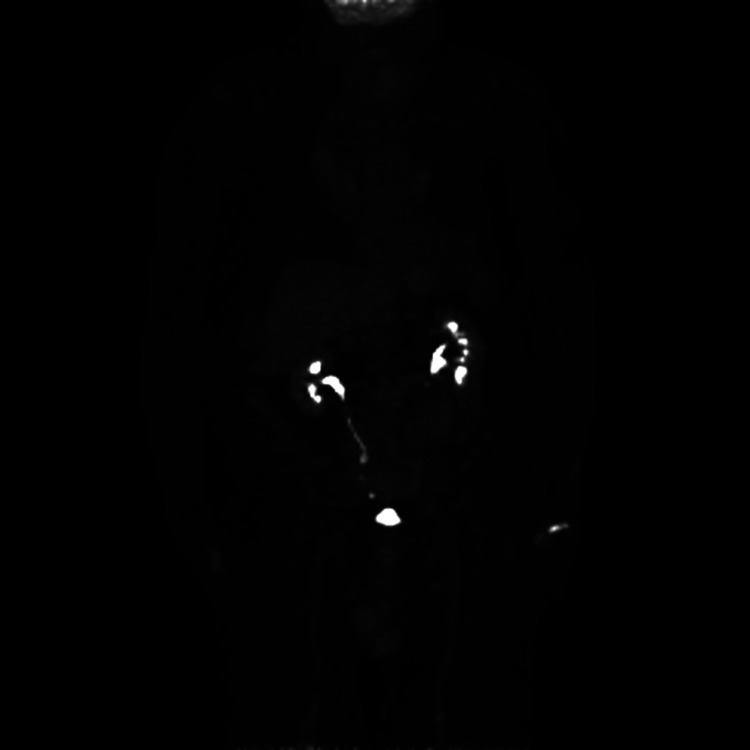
FDG PET/CT image revealing no evidence of malignancy or metastases. FDG: 18F-fluorodeoxyglucose; PET: positron emission tomography
